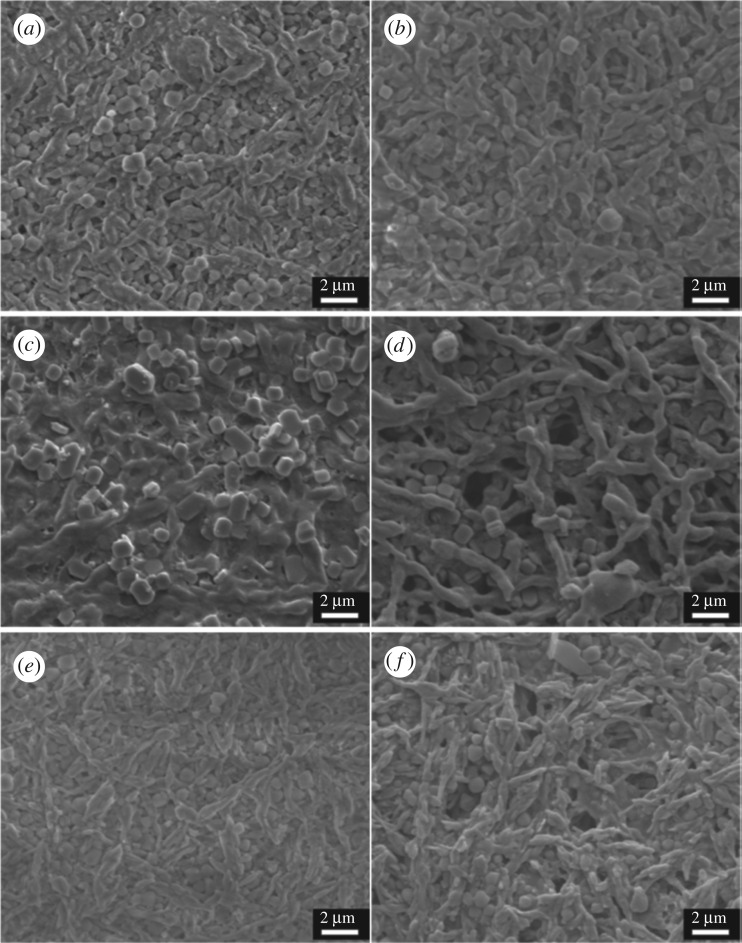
SEM images of (*a*) a NaA crystal-seeded HNM support before and (*b*) after a 2 min ultrasonic treatment; SEM images of (*c*) a silicalite-1 crystal-seeded HNM support before and (*d*) after a 2 min ultrasonic treatment; SEM images of (*e*) a NaY crystal-seeded HNM support before and (*f*) after a 2 min ultrasonic treatment.
